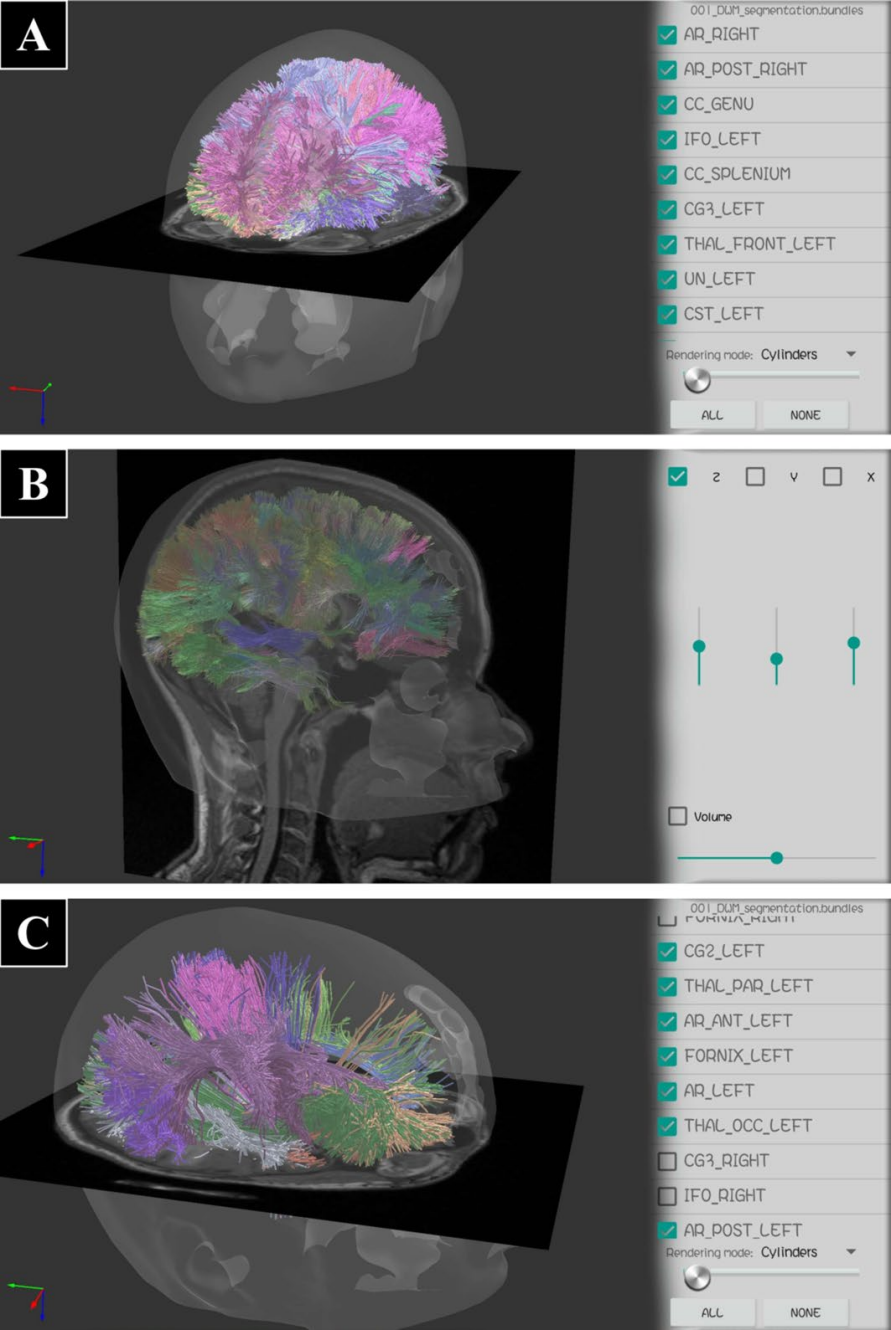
Example of brain data visualization of a healthy subject, consisting of a head mesh (semi-transparent), a brain MRI and a tractography dataset with labeled bundles (1% of the fibers displayed) (Dataset II). A An axial slice of the volume, all the bundles with cylinders, and the head mesh. B A sagittal slice, all the bundles with lines, and the head mesh. C An axial slice, some selected bundles with cylinders, and the head mesh
